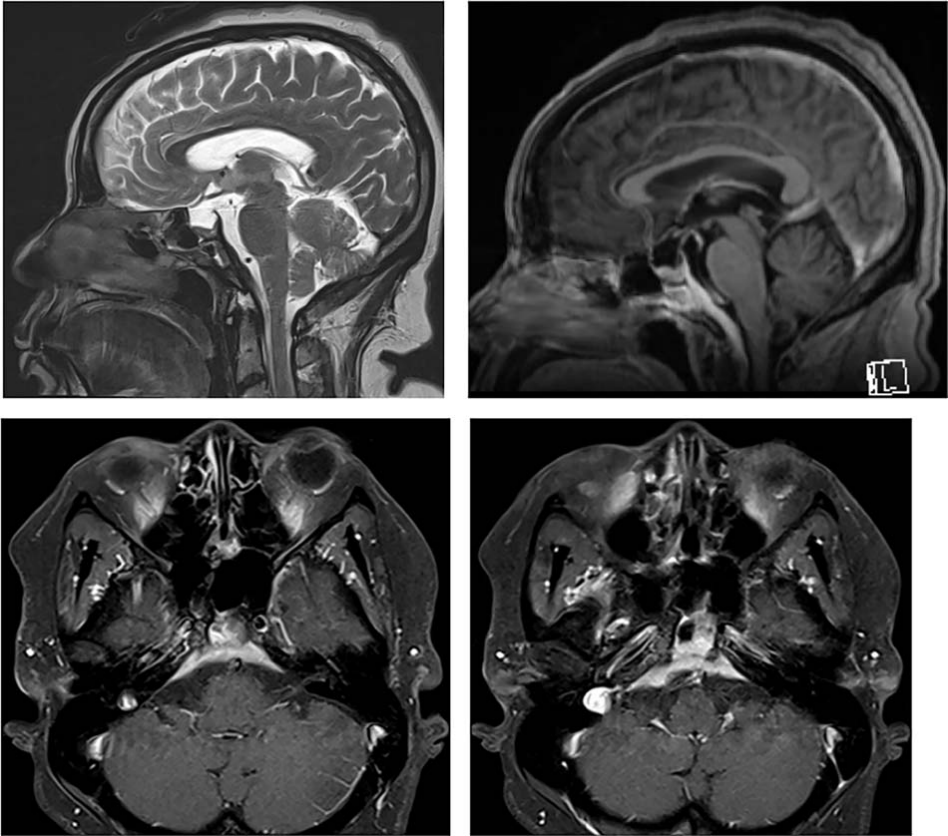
MRI of the head and orbits (top row: sagittal T2WI and sagittal T1WI post-contrast; bottom row: axial T1WI post-contrast at two levels [cranial to caudal]) showing increased retroclival dural thickening; the volume of fluid and mucosal thickening in the sphenoidal sinuses and clival cavity is reduced.
